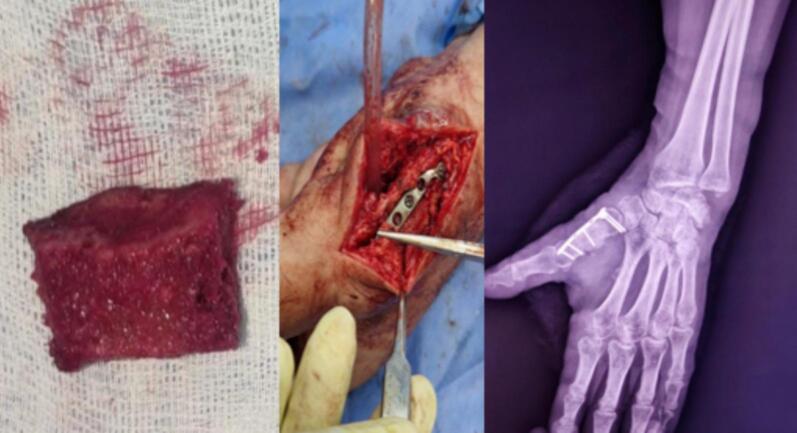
Intraoperative images and postoperative radiograph of the second stage of the Masquelet procedure.
